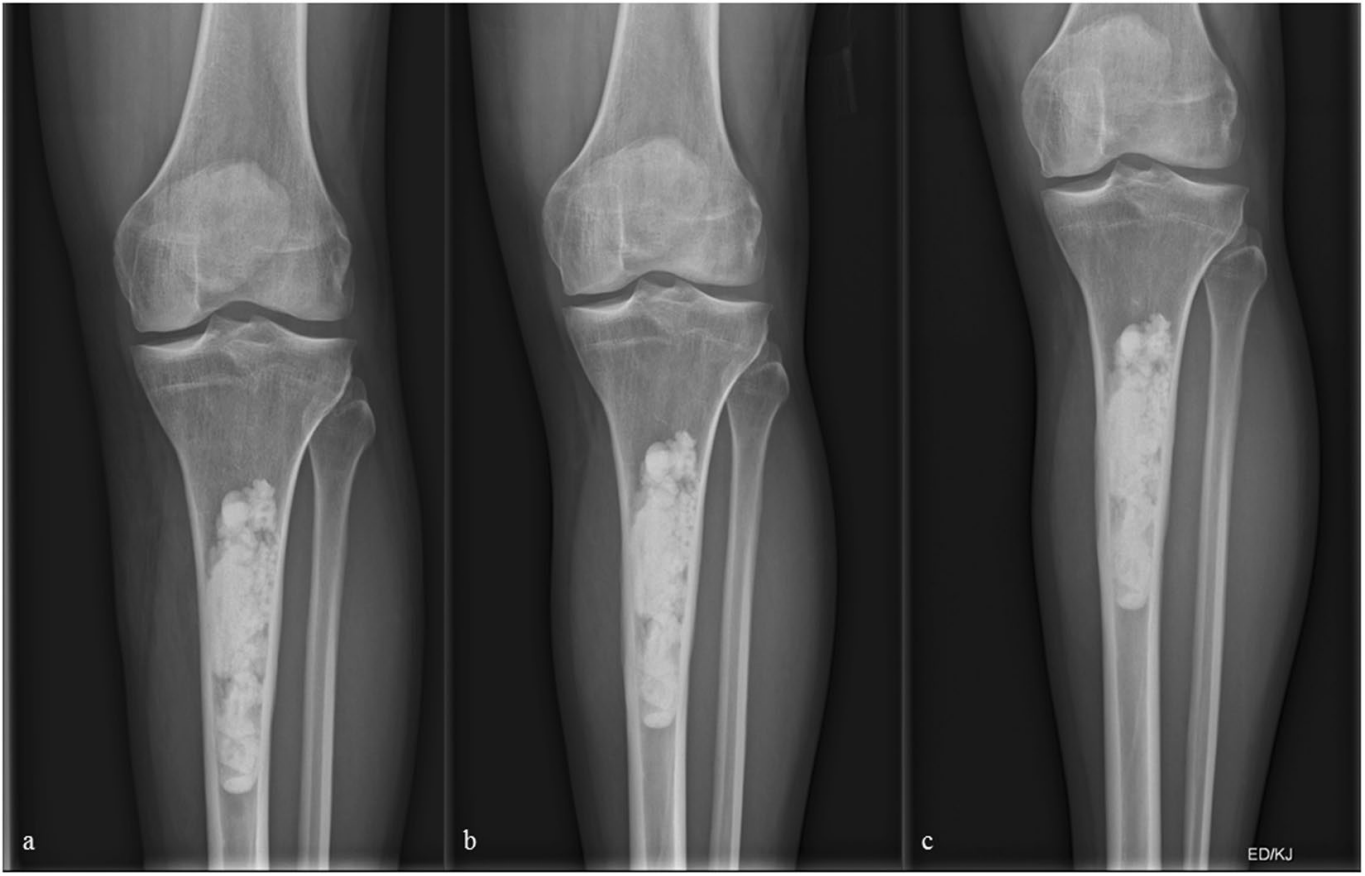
(a–c) X-ray of a 36 year-old male patient few days following curettage of a low-grade chondrosarcoma of the left proximal tibia. (b and c) Follow-up radiographs 7 and 13 months following index surgery showing integration but no resorption of the artificial bone graft substitute.
